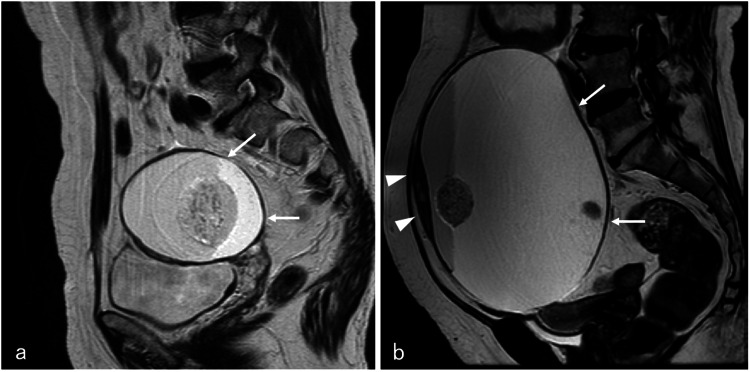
Ovarian tumor on MRI (a) MRI (T2-weighted imaging) performed 10 years ago revealed a left ovarian teratoma measuring 8 cm (arrows); (b) MRI (T2-weighted imaging) performed at our department showed a large cystic tumor measuring 17 cm, consisting of fat, fluid-fluid level, and a hair ball (arrows). The anterior wall of the cyst was slightly thick (arrowheads).
